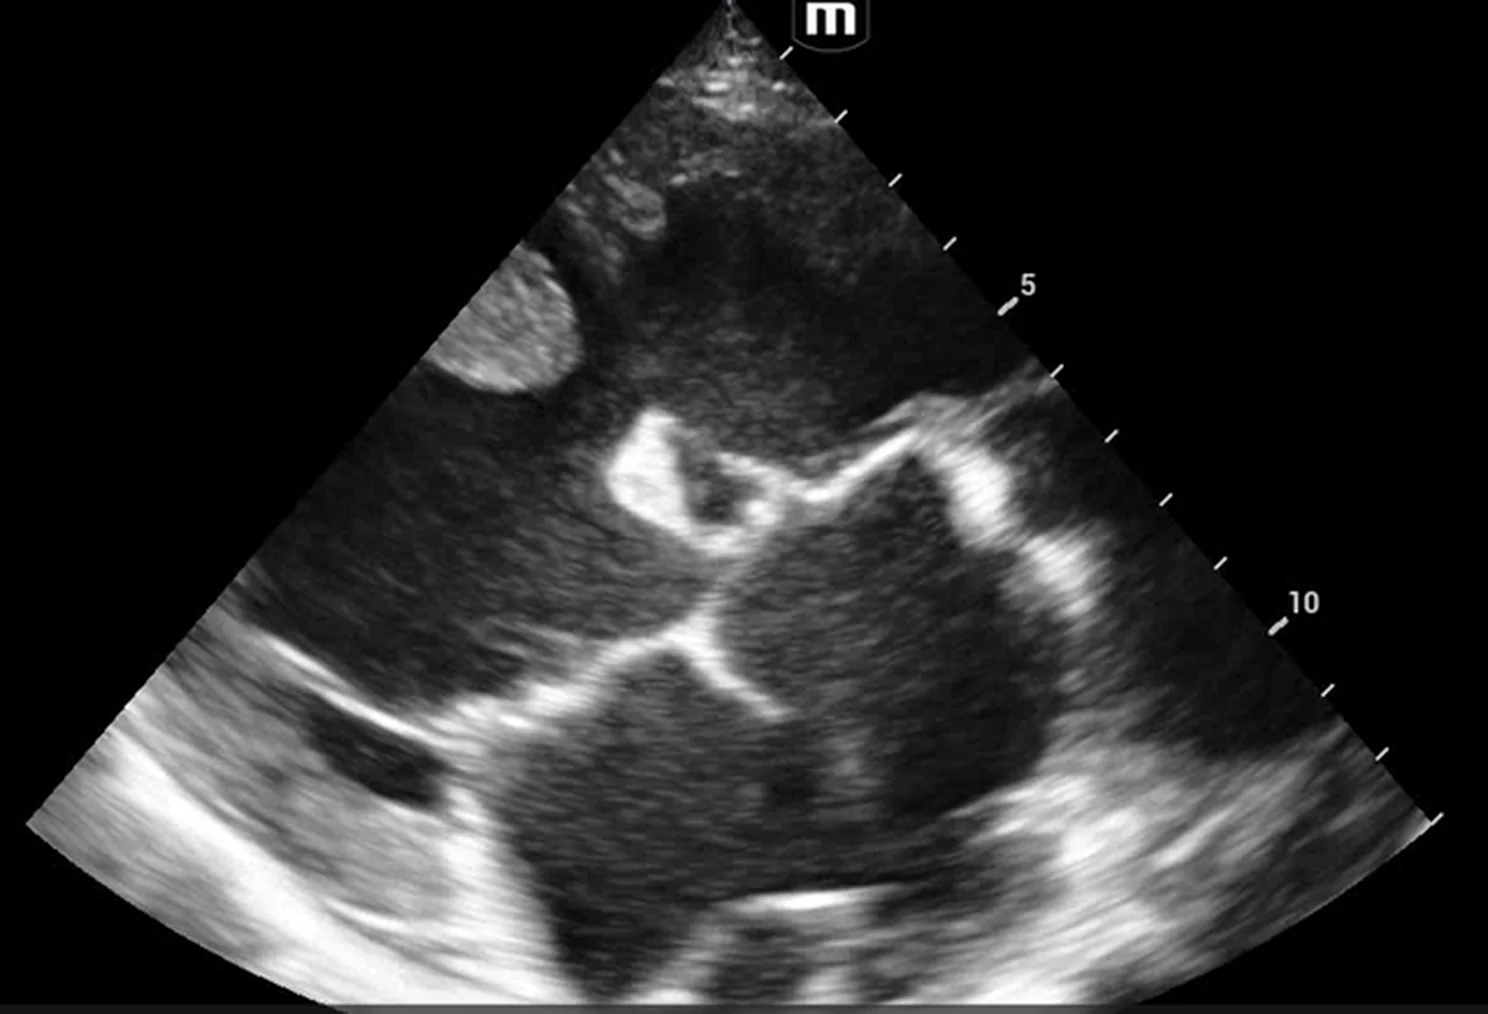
Modified four-chamber transthoracic echocardiographic view demonstrating large ventricular and atrial septal defects
